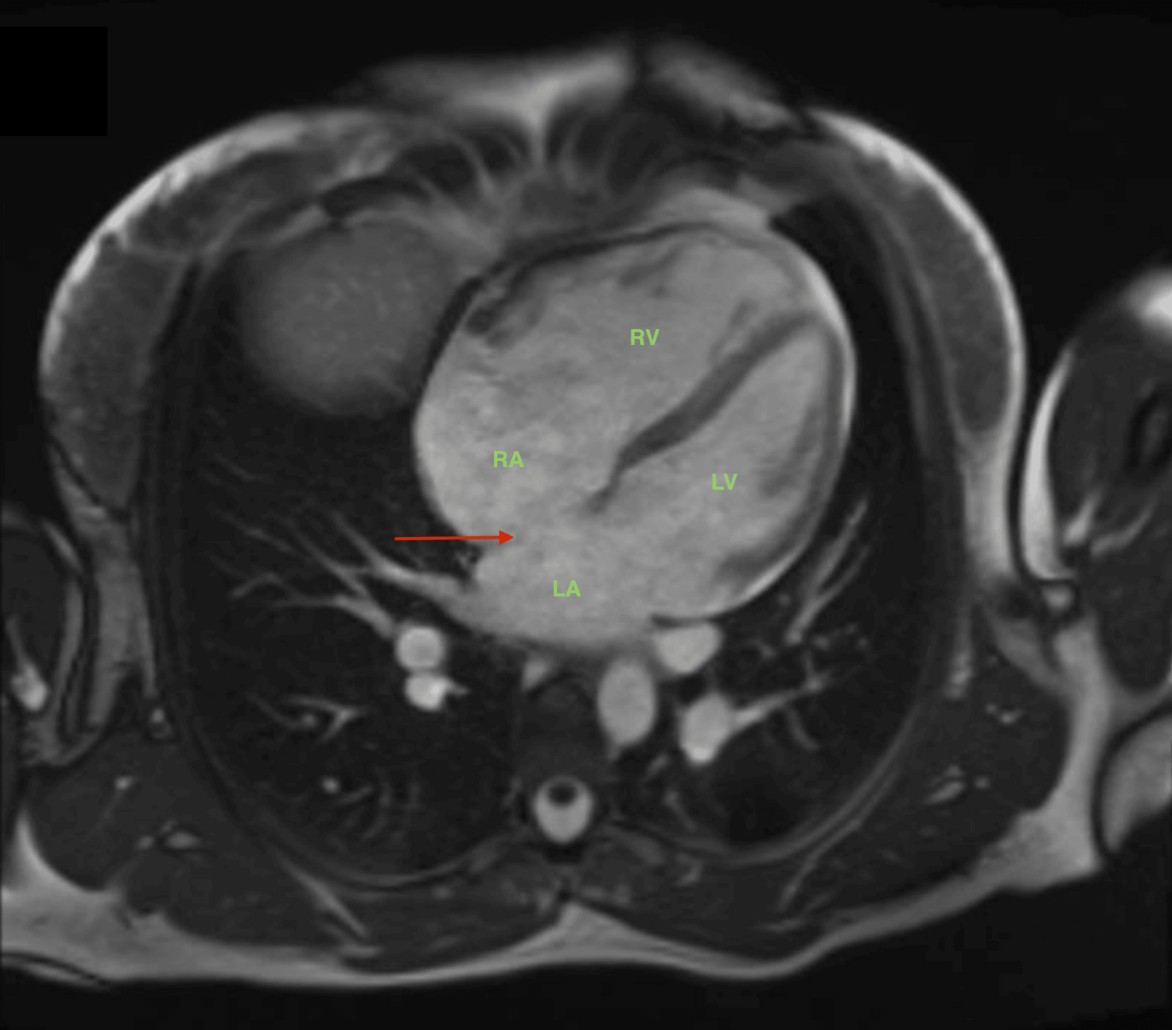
Preoperative cardiac MRI (transverse plane) demonstrating enlarged right cardiac cavities and a large atrial septal defect LA: left atrium; LV: left ventricle; RA: right atrium; RV: right ventricle Red arrow: atrial septal defect
